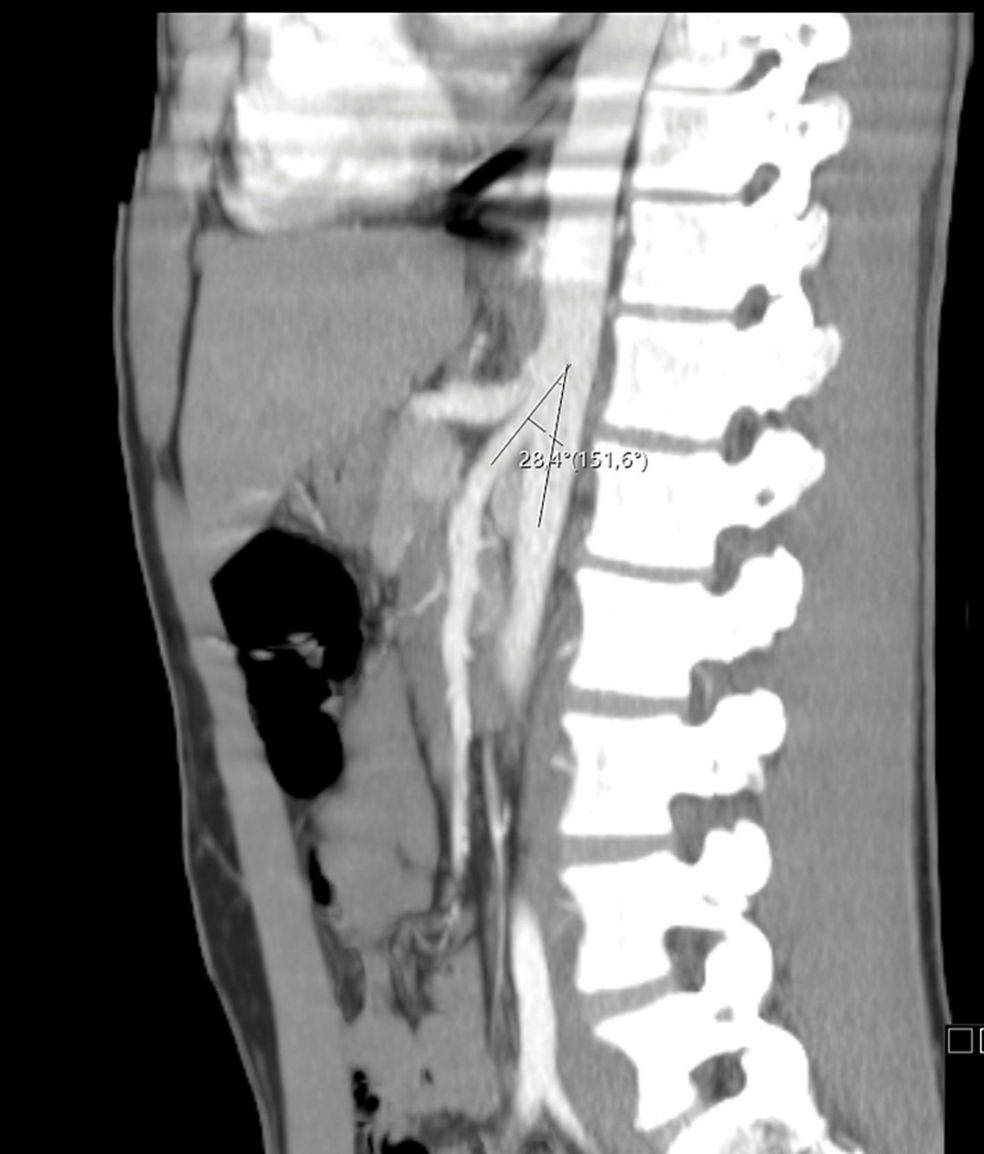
CT angiography followed by an acquisition in the excretory phase (CT urography) showing an angle between the SMA and aorta of 28.4°. CT: computerized tomography; SMA: superior mesenteric artery
